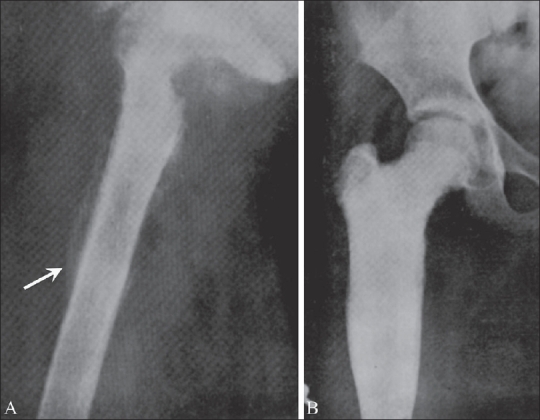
Ewing's sarcoma of the proximal femur. Pre-irradiation (A) oblique radiography shows an “onion-peel” appearance (arrow) with destruction of the marrow cavity. Post-irradiation (B), there is marked sclerosis and cortical thickening
